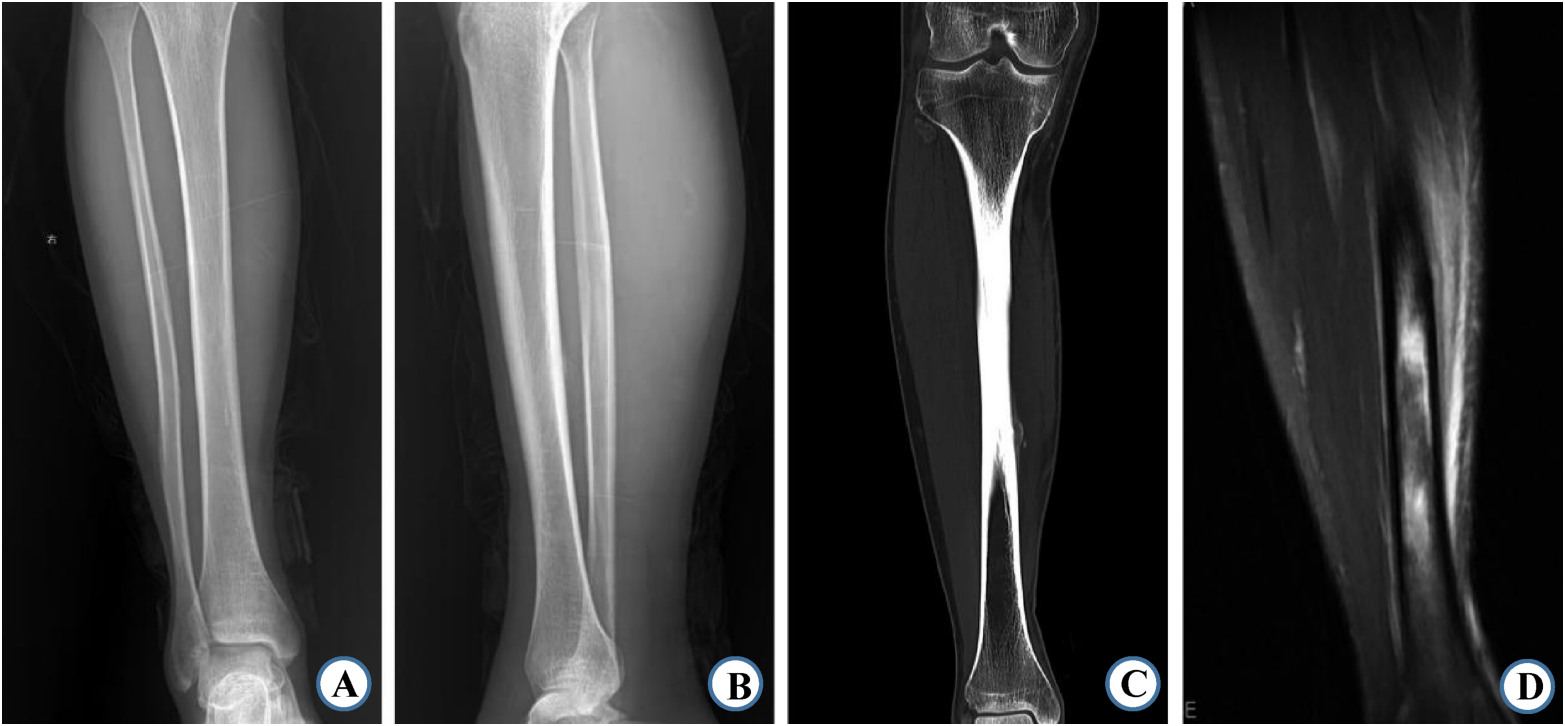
A 19-year-old male with a right tibial Type I stress fracture. (A,B) No significant abnormality observed on x-ray in the anteroposterior and lateral views. (C) CT scan shows localized thickening of the cortical bone in the middle segment of the right tibia. (D) MRI reveals a linear low-signal intensity area in the middle segment of the right tibia, surrounding patchy high-signal intensity areas on T2-weighted fast spin echo (T2FSE), and inner soft tissue edema.
